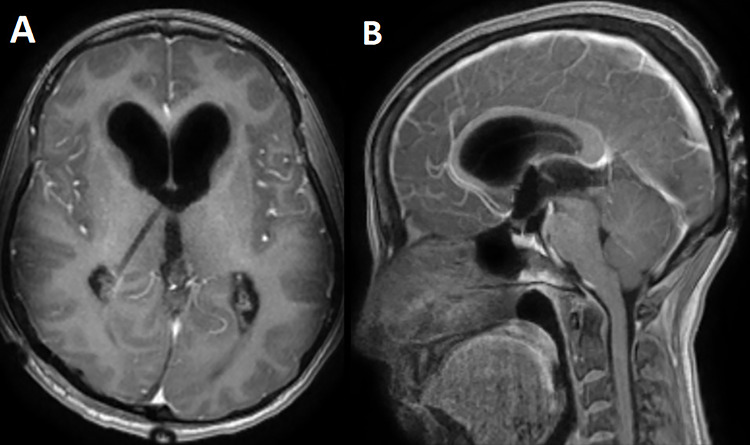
A postoperative day 1 T1 enhanced axial (A) and sagittal (B) MRI confirming complete surgical resection of the pineal tumor.
